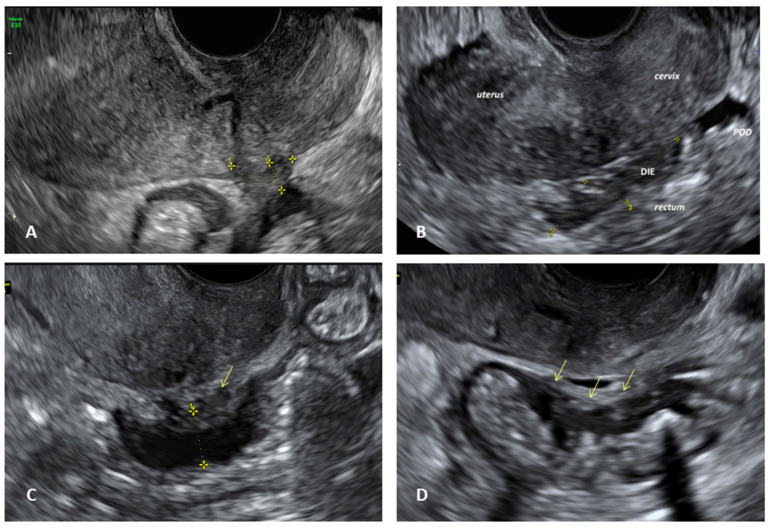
Transvaginal ultrasound (TVS) appearance of deep infiltrating endometriosis (DIE). (A) Utero-sacral ligaments (USL) hypoechoic nodule (dotted yellow lines); (B) DIE hypoechoic nodule involving rectum and uterine torus (POD: pouch of Douglas); (C) rectal endometriosis nodule (dotted yellow lines) and associated USL nodule (yellow arrow); (D) rectal endometriosis nodule (yellow arrows).
